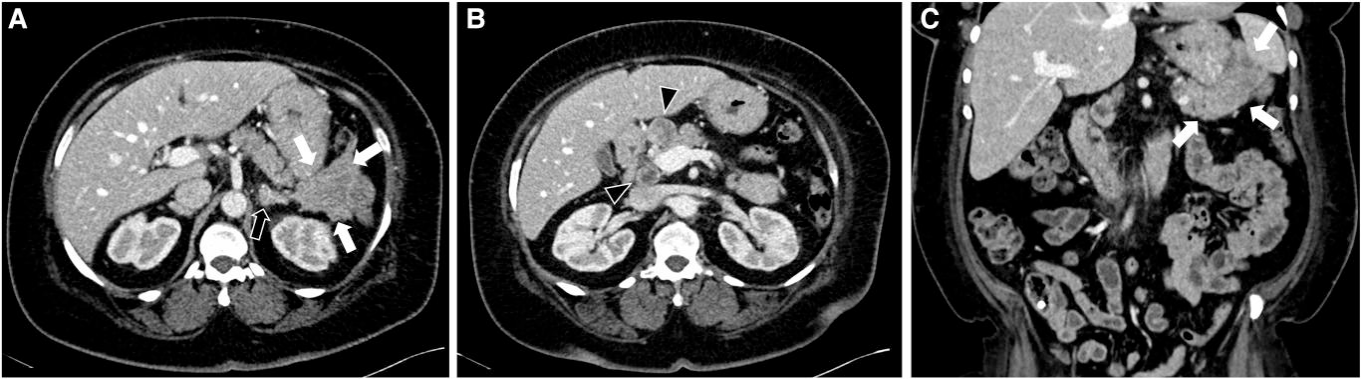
Contrast-enhanced abdomen CT in the axial (A, B) and coronal (C) planes demonstrates a lobulated, heterogeneously enhancing mass arising from the tail of the pancreas (white arrows, 2A, 2C) measuring 5.9 × 5.1 × 6.1 cm (AP, lateral, craniocaudal dimensions). Mass obliterates the splenic vein (not shown). Necrotic peripancreatic and periportal lymph nodes are present, measuring up to 2.6 cm in maximal dimension (black arrowheads, 2B). Nodular thickening of the left adrenal gland (black arrow, 2A) that demonstrated washout consistent with a lipid-poor adenoma.
